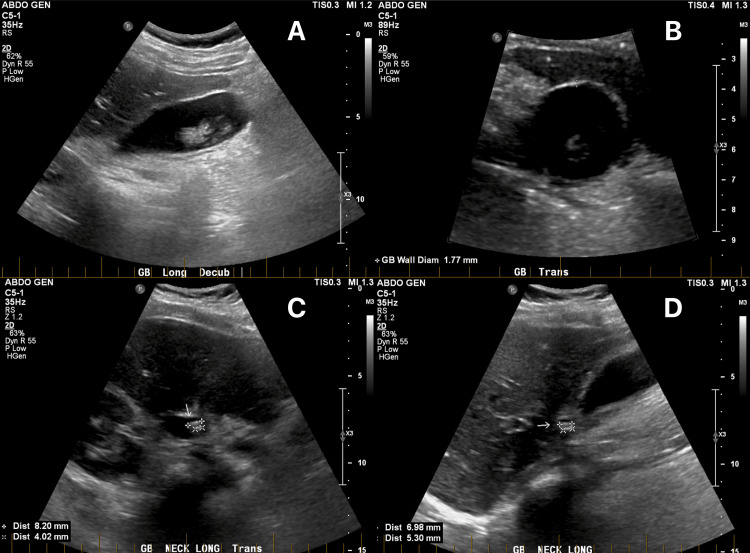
Ultrasound of the gallbladder on initial presentation. A: Longitudinal view. B: Thin gallbladder wall with a diameter of 1.77 mm. C, D: Gallstones impacted in the gallbladder neck.
